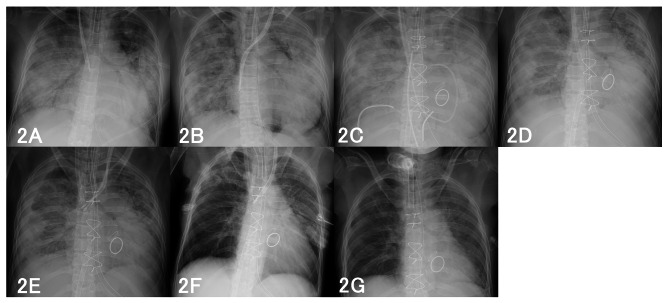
Chest radiographs during the course of treatment. A: on admission to ICU, B: preoperative radiograph, C: postoperative radiograph, D: before prone positioning, E: after prone positioning, F: before extracorporeal membrane oxygenation (ECMO) withdrawal, G: after respiratory weaning
